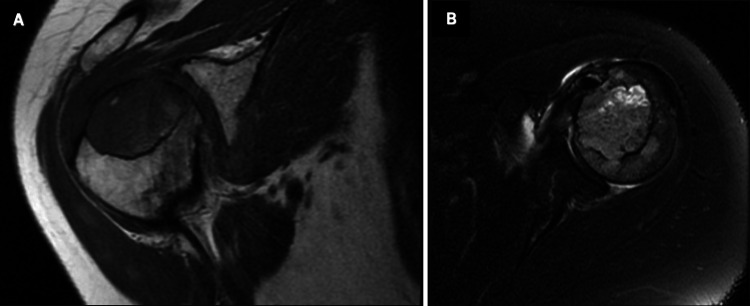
MRI of the right shoulder: coronary T1-weighted (A) and axial T2 fat-suppressed (B) views demonstrating a large humeral head lesion with cortical destruction of the greater tuberosity occupying greater than 75% of the proximal humerus.
